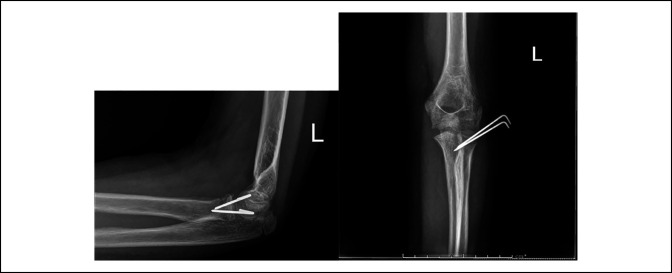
Images showing immediate postoperative fluoroscopic imaging of the elbow. Radiographs showing anteroposterior (A) and lateral (B) views of the left elbow after open reduction and pinning of the radial neck fracture.
